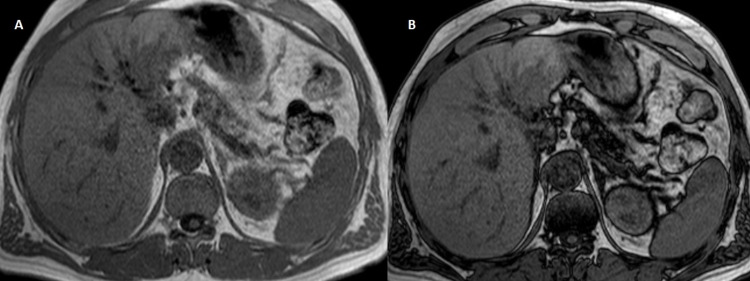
MRI shows a hypointense in T1w without signal loss in opposed-phase ({A} axial T1w GRE phase and {B} axial T1w GRE op-phase). T1w GRE: T1-weighted gradient-echo; T1w GRE op-phase: T1-weighted gradient-echo opposed-phase
